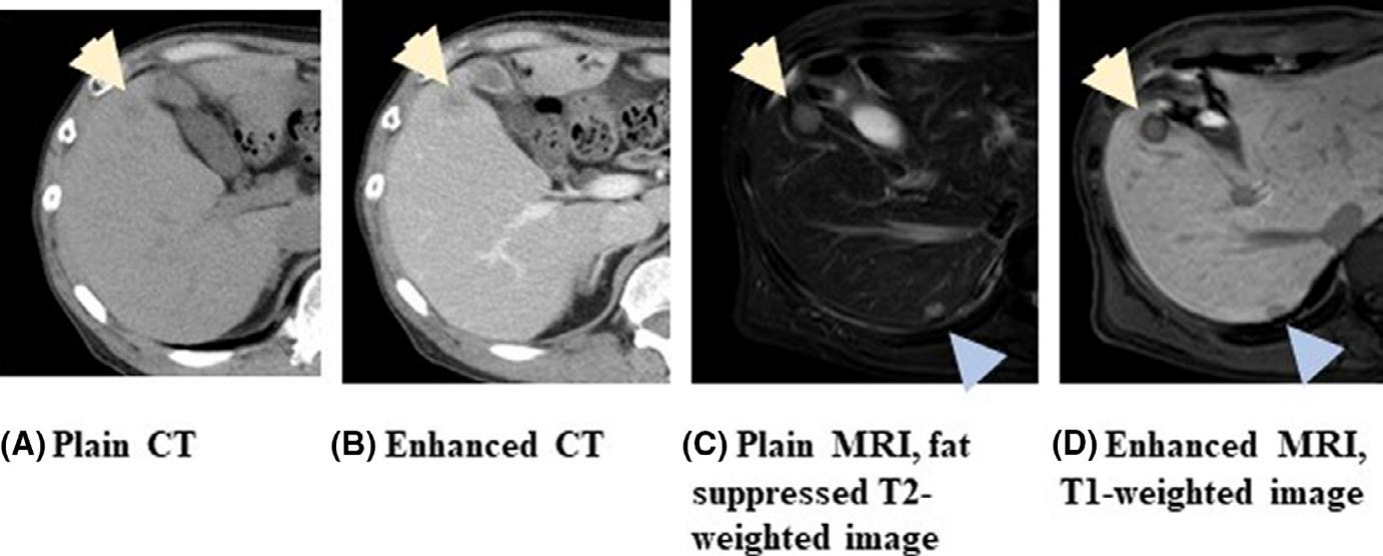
Representative CT images and MRI images to detect liver metastasis in the patients with colorectal cancer. (A) Plain CT, (B) Enhanced CT, (C) Palin MRI, fat suppressed T2‐weighted image, (D) Enhanced MRI, T1‐weighted image
